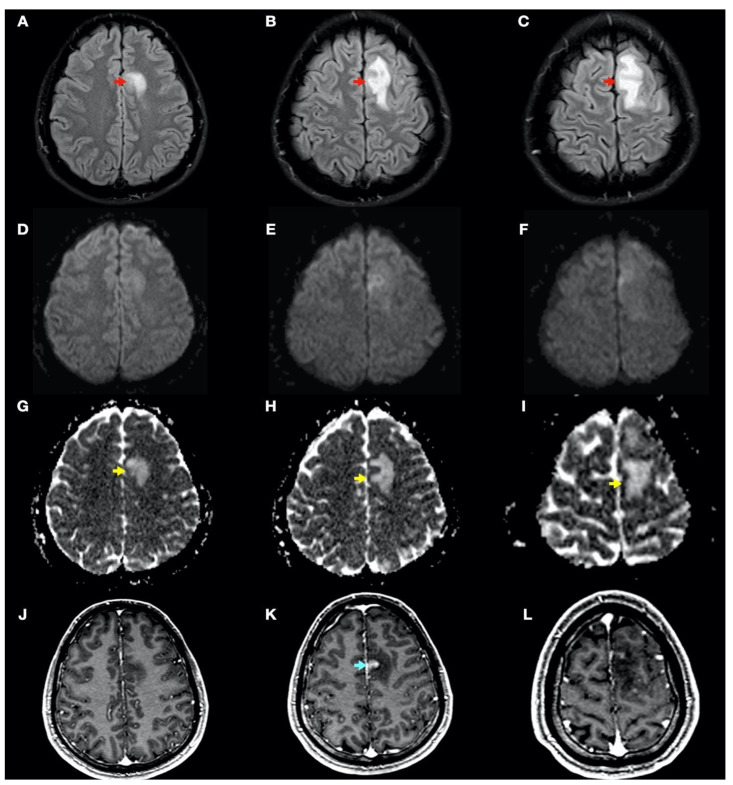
Brain magnetic resonance imaging (MRI): Images (A–I) from MRI 4 days after clinical onset; images (J–L) from MRI 5 days after clinical onset. Axial fluid-attenuated inversion recovery (FLAIR) images (A–C) show a left frontal cortico-subcortical hyperintense lesion (red arrows), also observed in other long-time repetition (TR) sequences (e.g., T2-weighted and proton density sequences). Axial diffusion-weighted imaging (DWI) (b1000) sequences (D–F) did not show a high signal intensity of lesions, whereas axial apparent diffusion coefficient maps (G–I) show a hyperintense lesion in this location (yellow arrows). (J–L) Axial gadolinium-enhanced 3D T1-weighted imaging (Gd T1WI) shows the presence of different areas of enhancement with irregular morphology (blue arrow), not suggestive of tumor enhancement.
